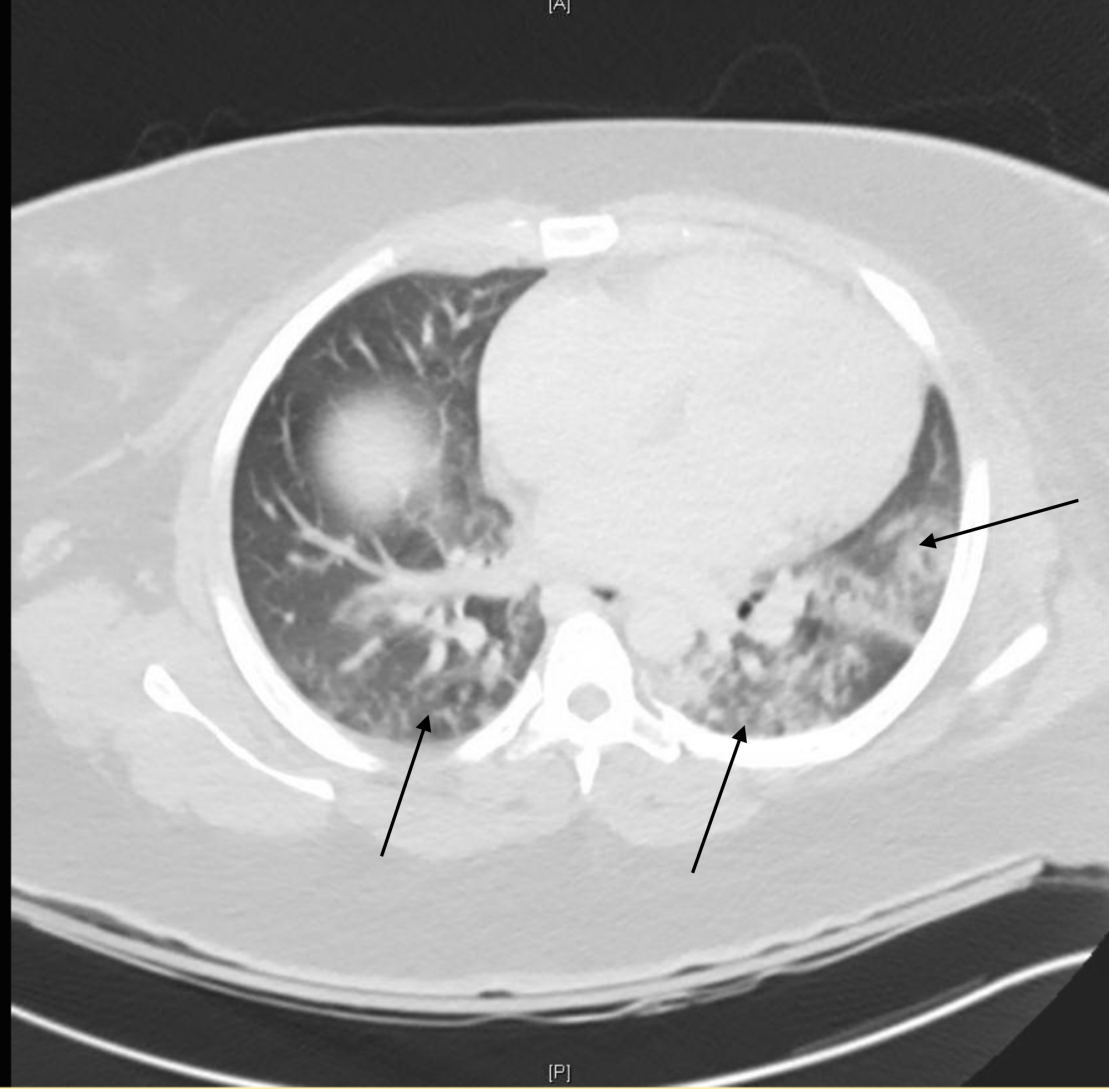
Computed tomography (CT) scan of the chest showing bilateral patchy opacities
